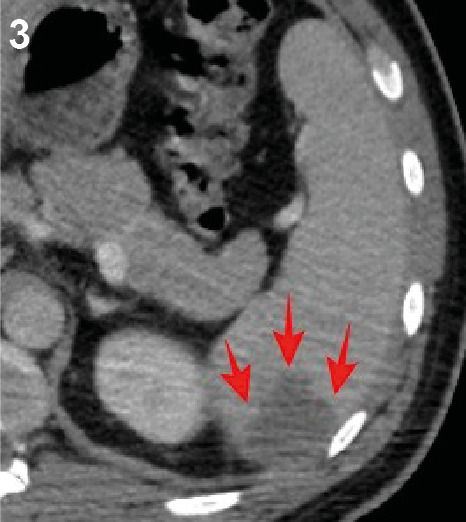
Venous phase contrast-enhanced computed tomography showing the infarct area in the spleen (red arrows).
